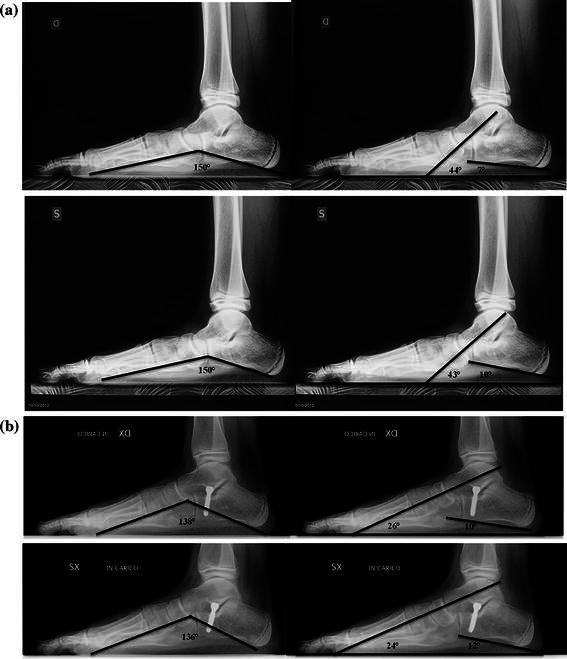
X-ray at weight-bearing of the same patient in Fig. 3 with measurements of Costa-Bartani angle (*left*) and talar inclination and calcaneal pitch angles (*right*). a pre-SESA, b post-SESA
